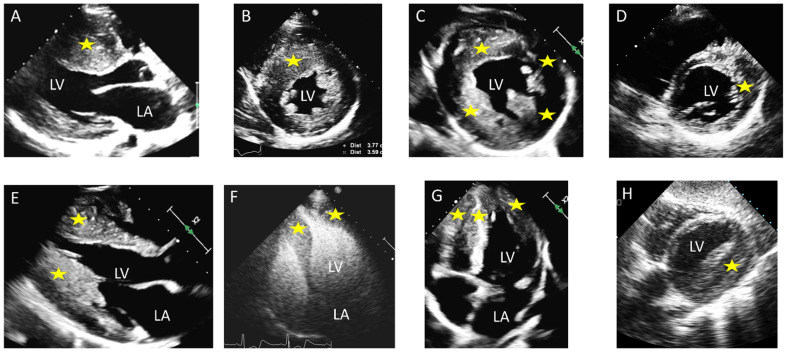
Different distribution and location of hypertrophy in hypertrophic cardiomyopathy (HCM) (asterisks): (A) (parasternal long axis) and (B) (parasternal short axis) septal hypertrophy; (C) (parasternal short axis) concentric hypertrophy; (D) (parasternal short axis) lateral hypertrophy; (E) (parasternal long axis) and (F) (contrast echocardiography, apical four-chamber) apical hypertrophy; (G) (apical four-chamber) left ventricular (LV) and right ventricular apical hypertrophy; (H) (subcostal view) lateral hypertrophy. LA: left atrium.
